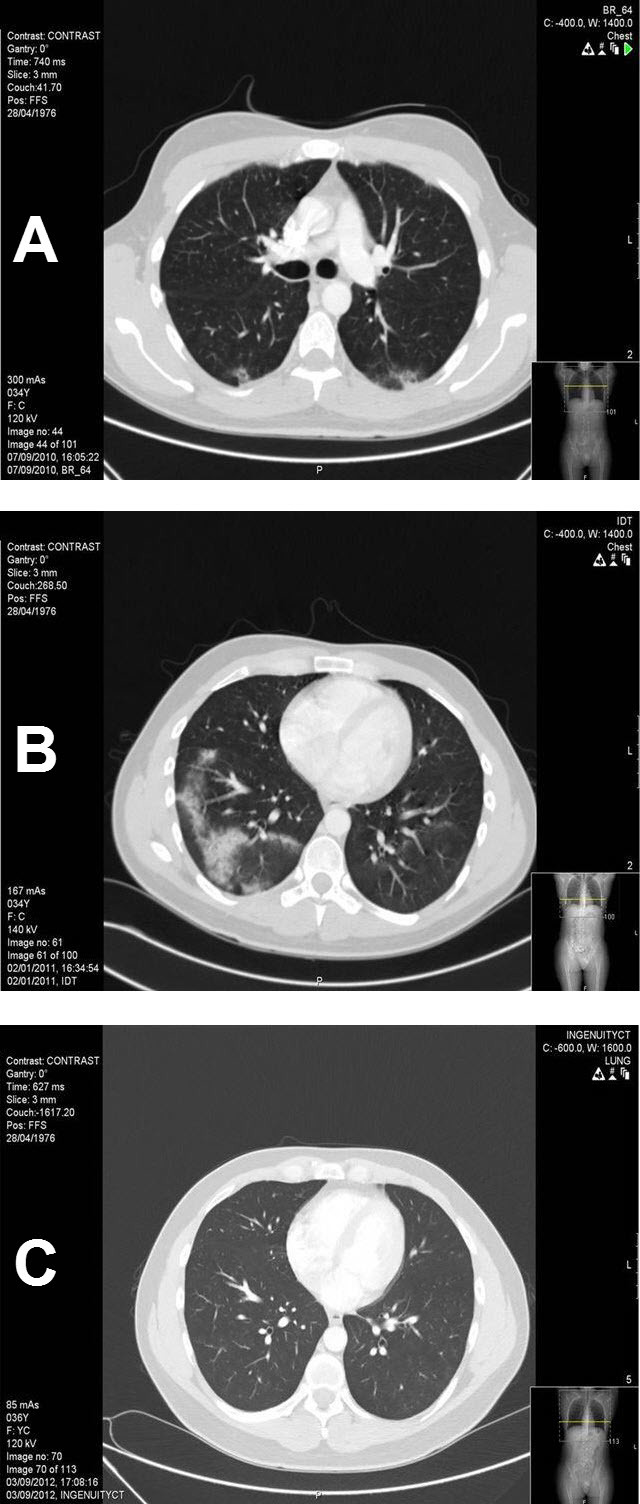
A CT Scan Following Completion of BEP Regimen (cumulative bleomycin dose 240 units) at 2 Months (A), 5 Months (B), 10 Months (C). A) 2 months: Interstitial alveolar infiltrates in the posterior, hanging parts of the right and left lower lobes; B) 5 months: Alveolar shadows in various sizes confined to both lung bases, more on the right side but also on the right middle and upper lobes—clear worsening compared to 1A; C) 10 months: Clear regression and improvement in the right lower and right middle lobes.
